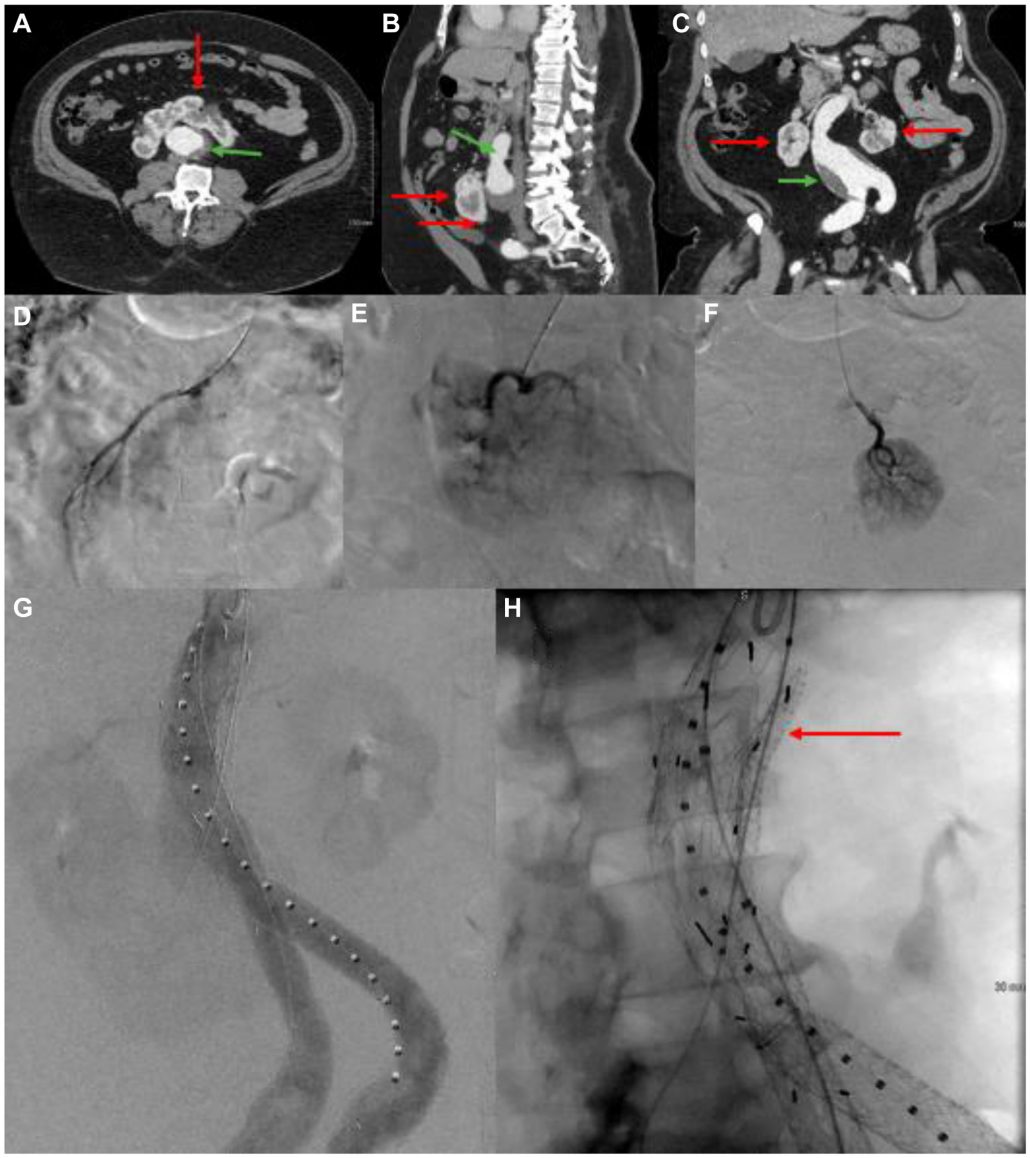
A-C, Computed tomography (*CT*) imaging of abdominal aortic aneurysm (*AAA*) (*green arrow*) and horseshoe kidney (*HSK*) (*red arrow*); D, Right renal artery; E, Anterior renal trunk artery; F, Left renal artery; G, Bifurcated endograft, aortic cuff, renal snorkel (*red arrow*), iliac extension, and flared limbs; H, Type II endoleak vs gutter leak.
